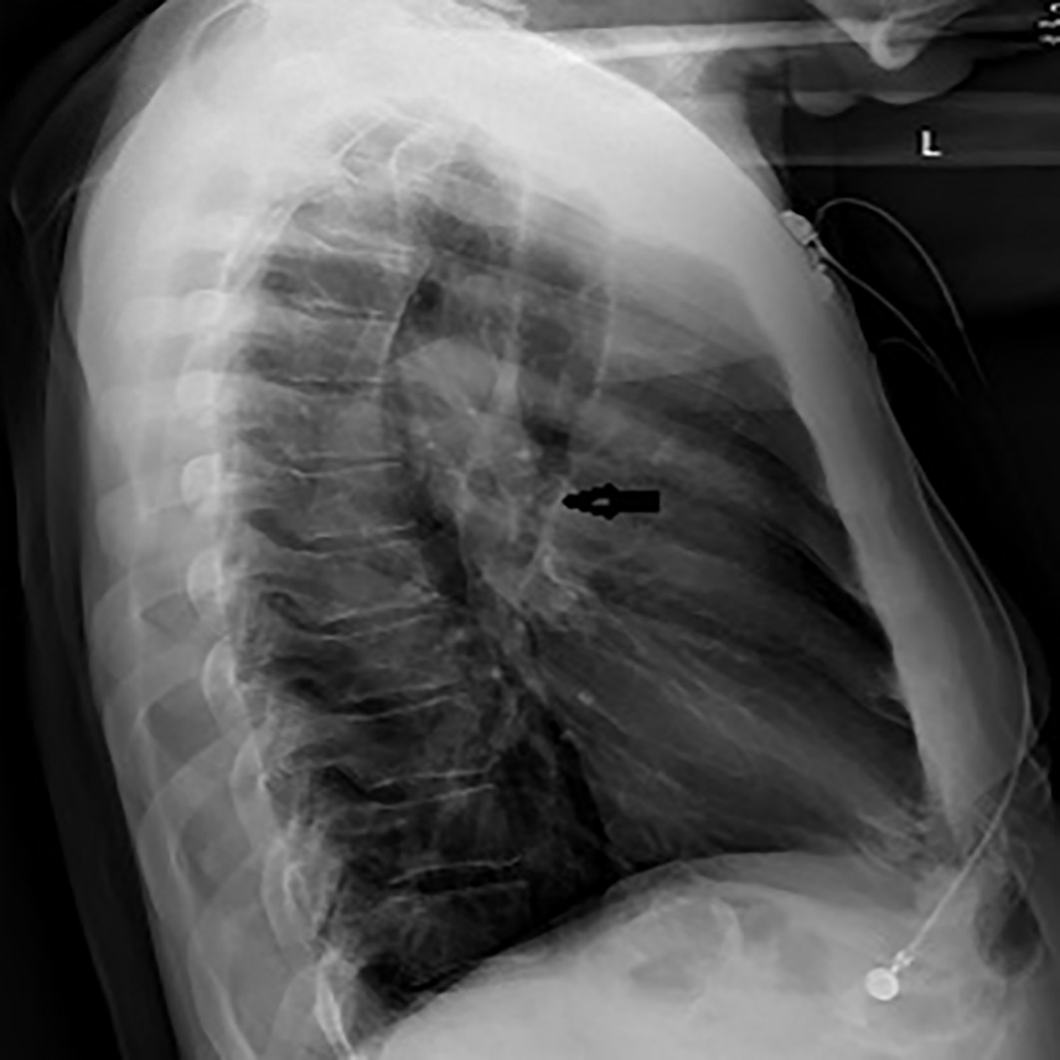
Chest X-ray (lateral) view showed debris in a dilated thoracic esophagus with fluid level (black arrow).
